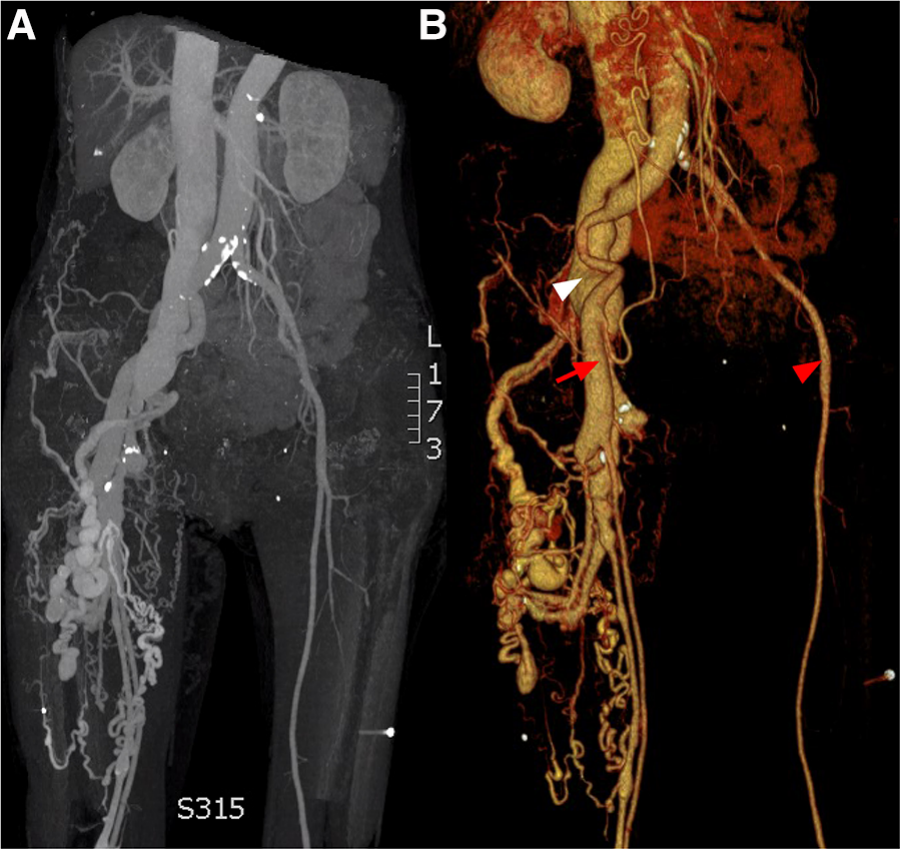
CT angiogprahy: (A) coronal section, (B) 3D reconstruction. Normal femoral artery (red arrow head), dilated femoral artery (red arrow) and aneurysmal dilatation of the iliac vein (white arrow head).
